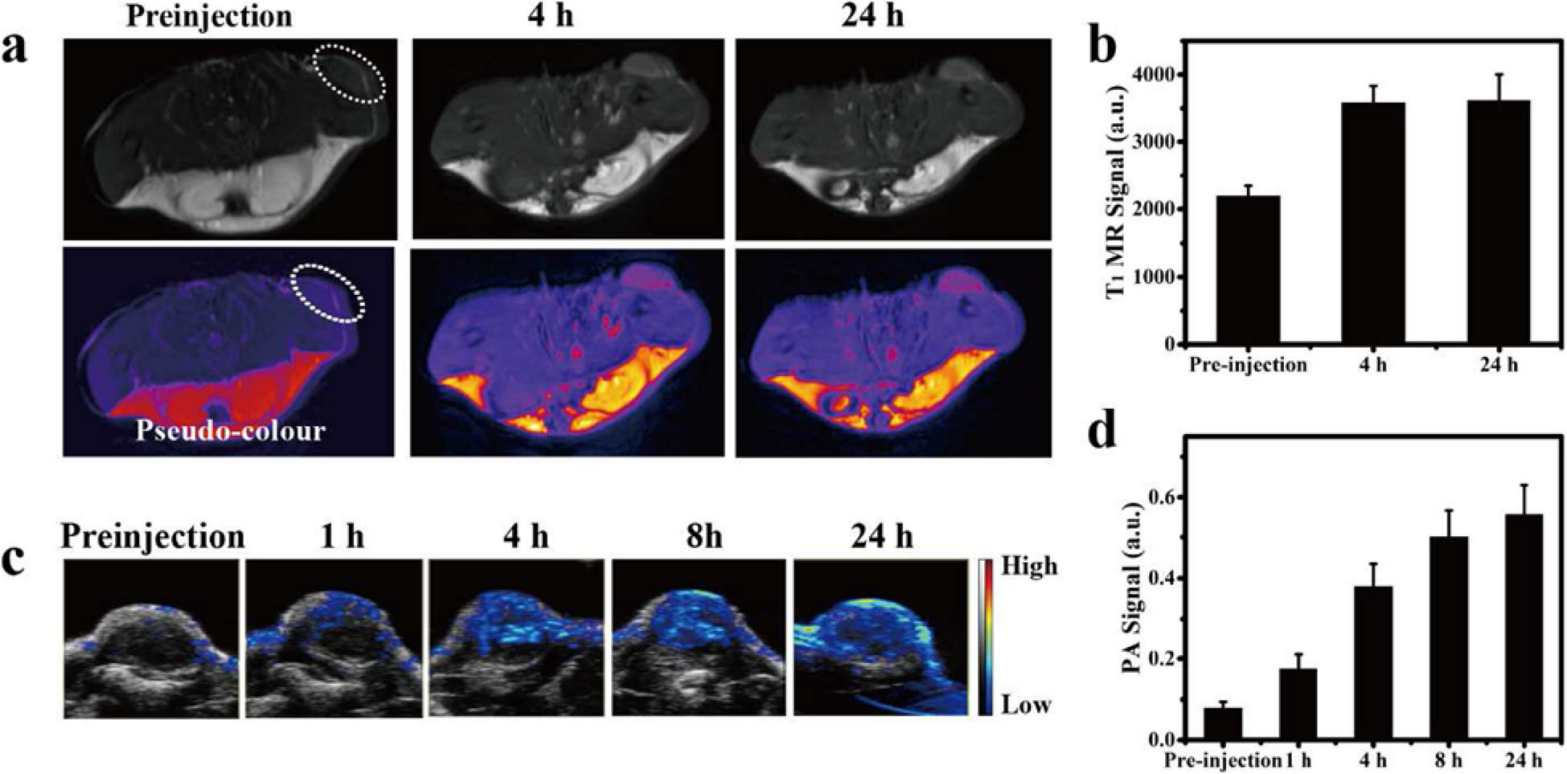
MRI and PAI performance in vivo [25]
